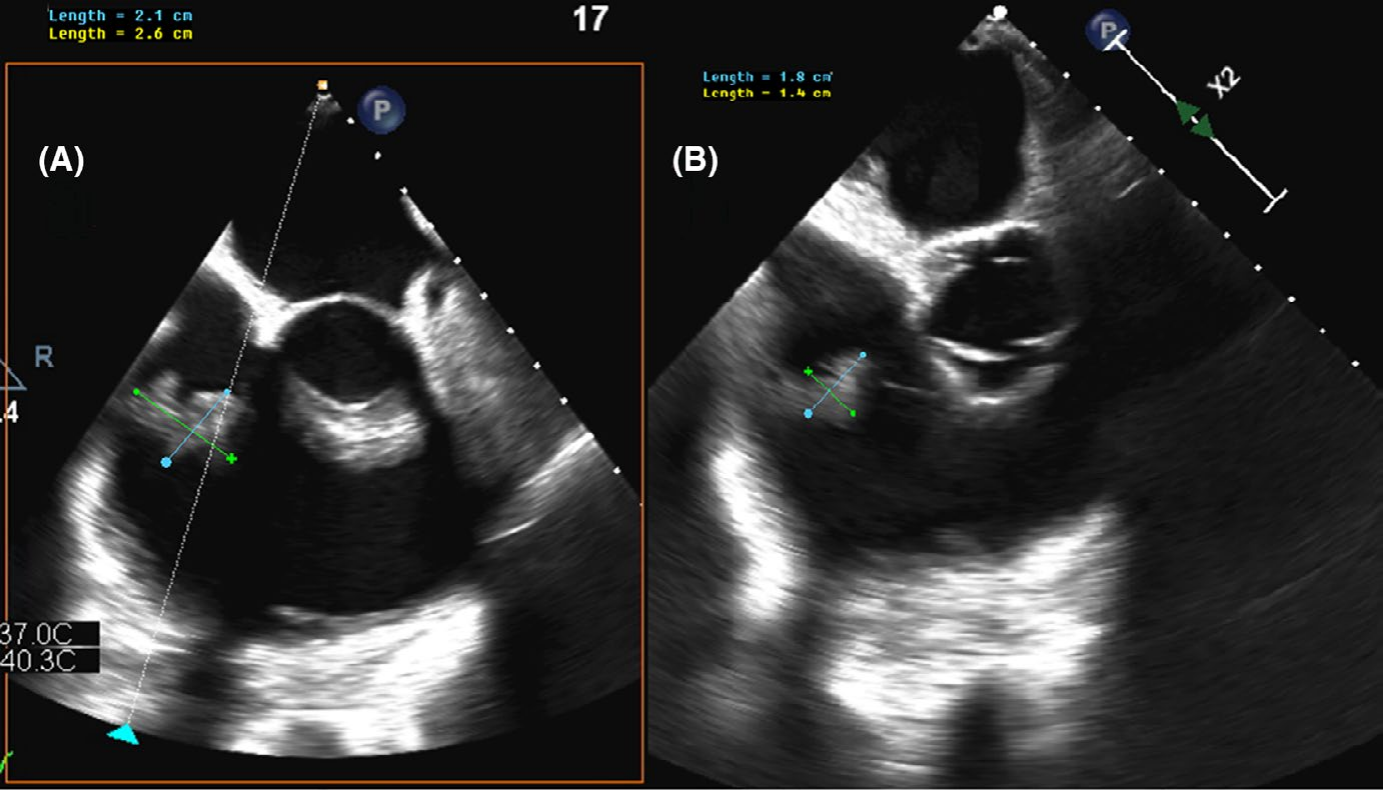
Mid‐esophageal short‐axis transoesophageal echocardiogram images demonstrating the tricuspid valve vegetation pre A, and post B, AngioJet debulking, with a greater than 50% reduction in size
